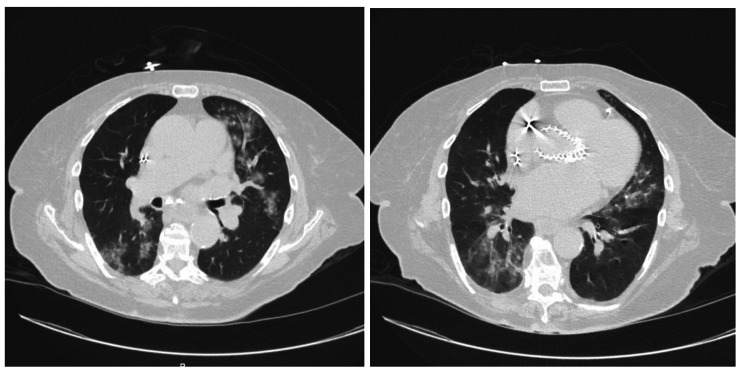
75 year old female with history of hyperlipidemia presenting with nausea and generalized weakness. Axial CT images in lung window demonstrate bilateral multifocal nodular and patchy ground-glass opacities consistent with infectious pneumonia
